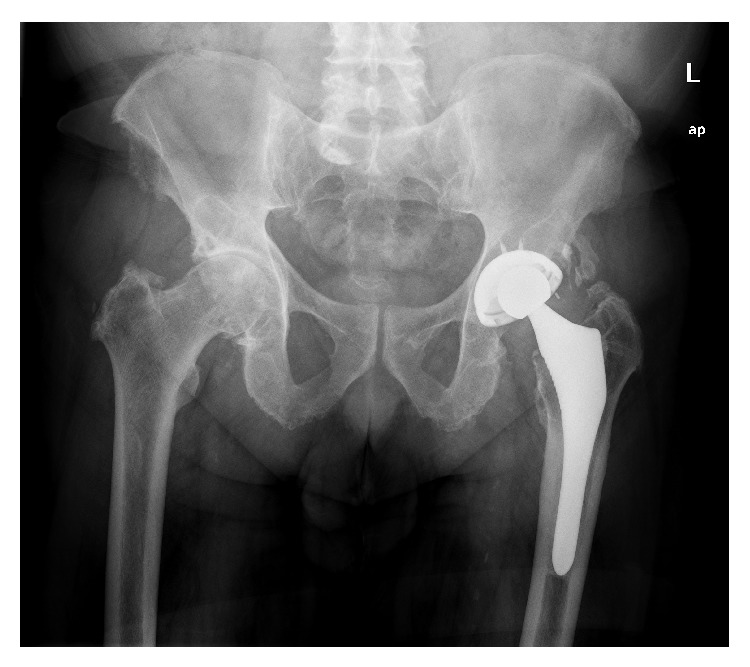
X-ray image of patient with follow-up at 12 y. Excessive polyethylene wear. Osteolysis in metaphyseal of femur.
